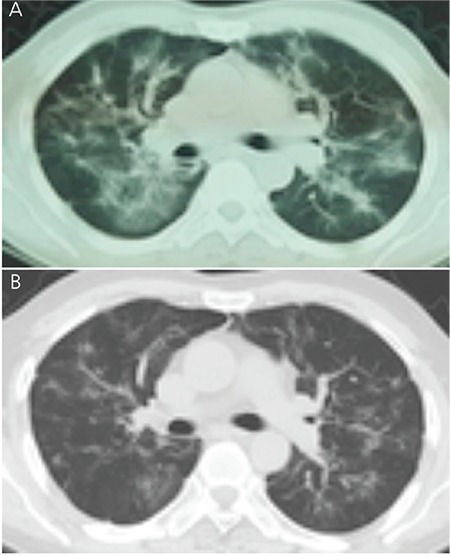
A) Chest computed tomography (CT) shows bilateral diffuse subpleural nodules, interlobular septal thickening, reticulation, and peribronchial ground glass opacities in both lungs. B) Chest CT scan 8 months after the switch to prednisone and nilotinib: lung abnormalities were decreased.
